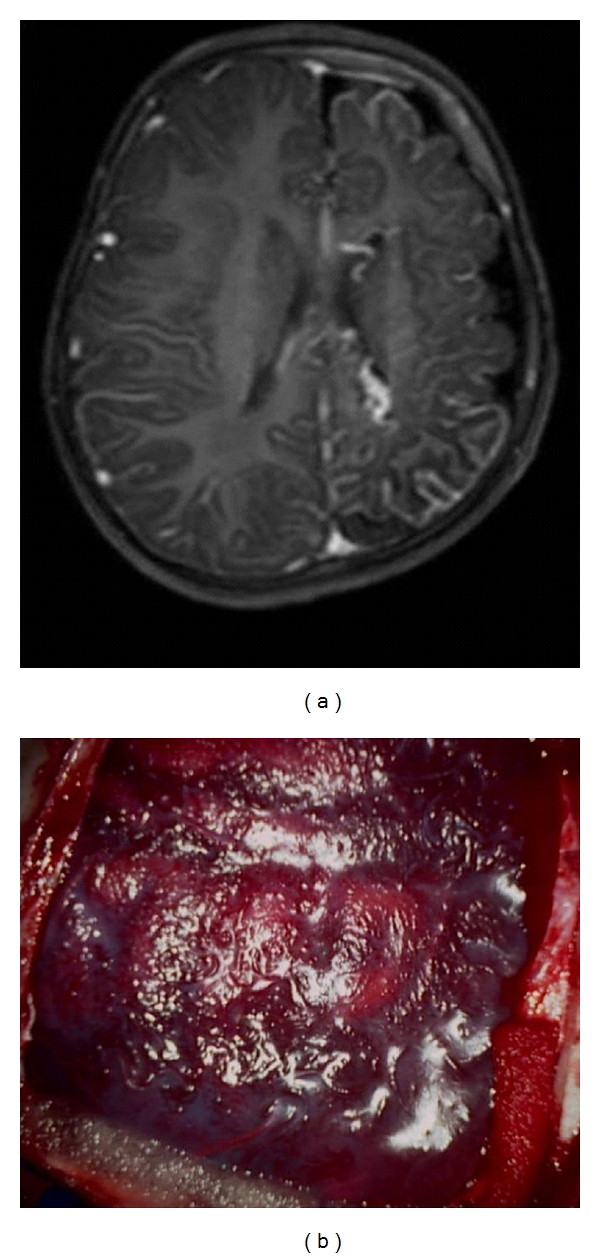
(a) T1W Axial gadolinium MRI image of a patient with Sturge Weber disease showing left hemispheric leptomeningeal angiomatosis, (b) intraoperative photograph.
